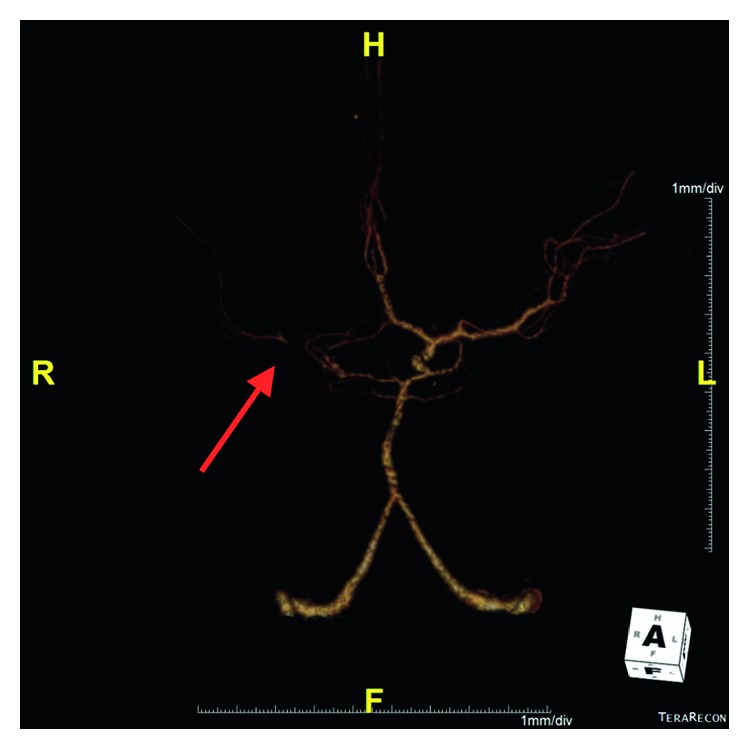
3D reconstruction of the CTA showing poor filling and blood flow in the right MCA.
